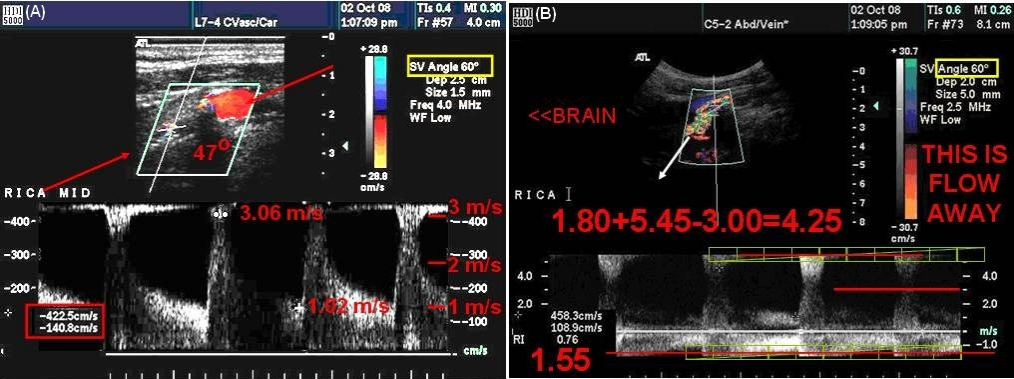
Severe Stenosis Misclassification. A. Misalignment of the Doppler cursor, yielding an elevated angle measurement, results in an EDV > 1.4 m/s, indicating a severe stenosis within this stent. Proper cursor alignment would result in EDV = 1.02 m/s, indicating a moderate stenosis. Note the aliased systolic peak extending to the 100 cm/s value. After correcting for aliasing and for angle misalignment, the correct systolic velocity is 3.8 m/s. B. Faced with a bidirectional waveform, the examining sonographer measured the reversed (+) portion of the waveform (indicating arterial flow from brain to heart), measuring the EDV as 1.09 m/s and indicating moderate stenosis, rather than the correct 1.55 m/s value indicating severe stenosis. They also measured a PSV of 4.58 m/s rather than the correct 4.25 m/s value.
